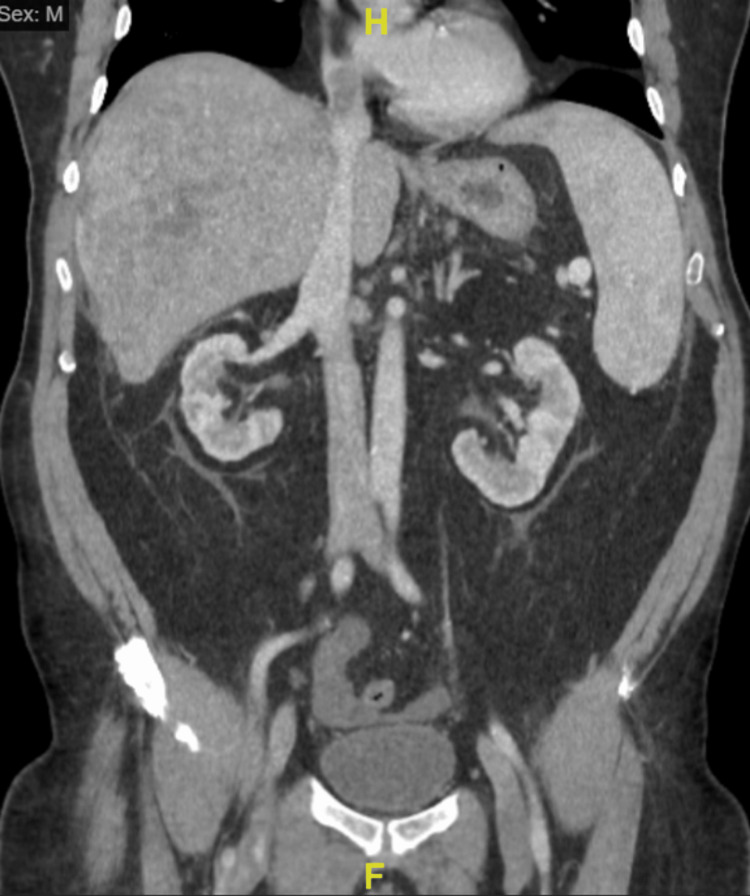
CT Abdomen and Pelvis Without Contrast Performed at Hospital Admission. Multiple hepatic lesions with findings concerning for tumor thrombus within the right hepatic and main portal veins with extension to the inferior vena cava and right atrium. Multiple hepatic lesions, the largest within the right hepatic lobe measuring 12.6x2.8x10.6 cm, exhibiting heterogeneous enhancement. In the setting of cirrhosis, this is concerning for multicentric hepatocellular carcinoma, and metastatic disease is difficult to exclude. Sequela of portal hypertension including splenomegaly, dilated main portal vein, and trace ascites. Colonic diverticula without evidence of diverticulitis. Mild prostatomegaly. Yellow "H" indicates the direction of head, while yellow "F" indicates the direction of feet.
